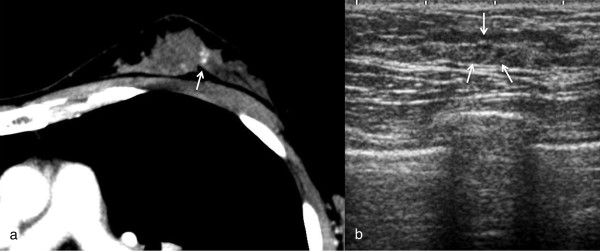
MDCT showed clustered enhancement on the ipsilateral breast. Targeted sonography detected a hypoechoic lesion, but it was difficult to differentiate the surrounding tissue. An excisional biopsy was performed at the same time as breast lumpectomy to obtain a definitive diagnosis. The pathological diagnosis was columnar cell hyperplasia.
